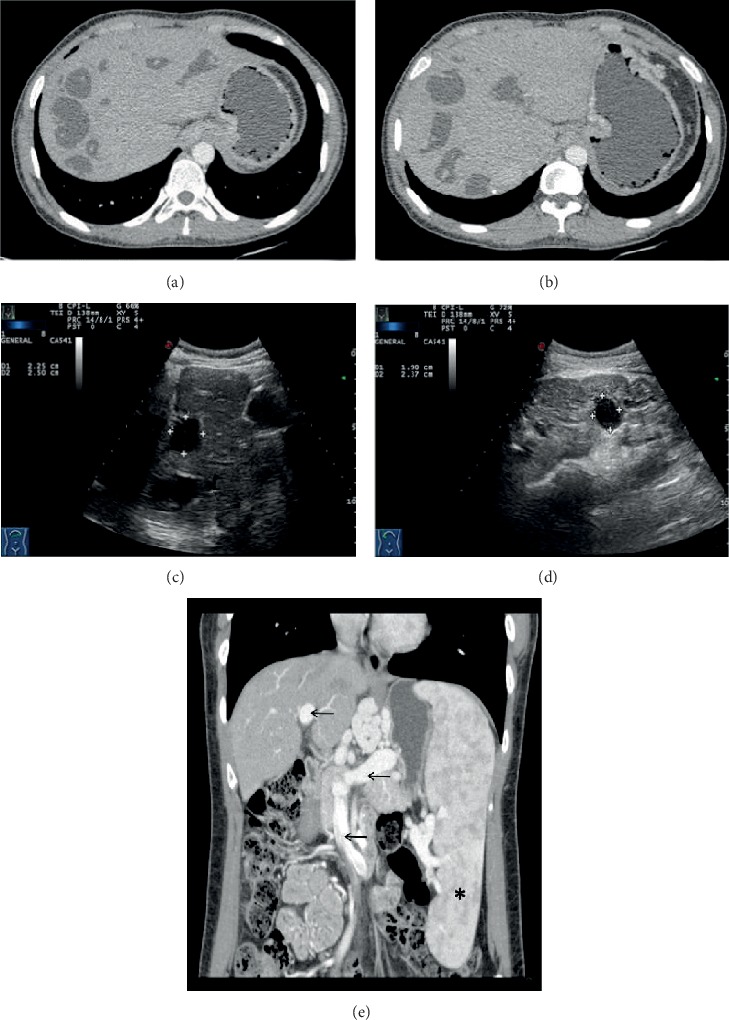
Portal vein CT venography- (CTV-) enhanced scan (a, b) and abdominal ultrasound (c, d) of P5 suggested dilation of the intrahepatic bile ducts with the maximum diameter of 30 mm in CTV. The liver was normal in size with smooth capsules and an unevenly distributed rough echo. There are multiple well-defined hypoechoic mass lesions in the liver and bilateral kidneys. The comet's tail echoes diffused in the kidney ultrasound suggesting multiple calcifications. The spleen was not shown due to splenectomy. Three-dimensional reconstruction of the portal vein CTV-enhanced scan of P6 (e). The maximum diameter of the portal venous trunk was 19 mm (arrow). The spleen was markedly enlarged (asterisk), and intrahepatic portal vein branches were dilated, including the superior mesenteric vein (arrow), splenic vein (arrow), esophageal and gastric veins, and umbilical vein.
